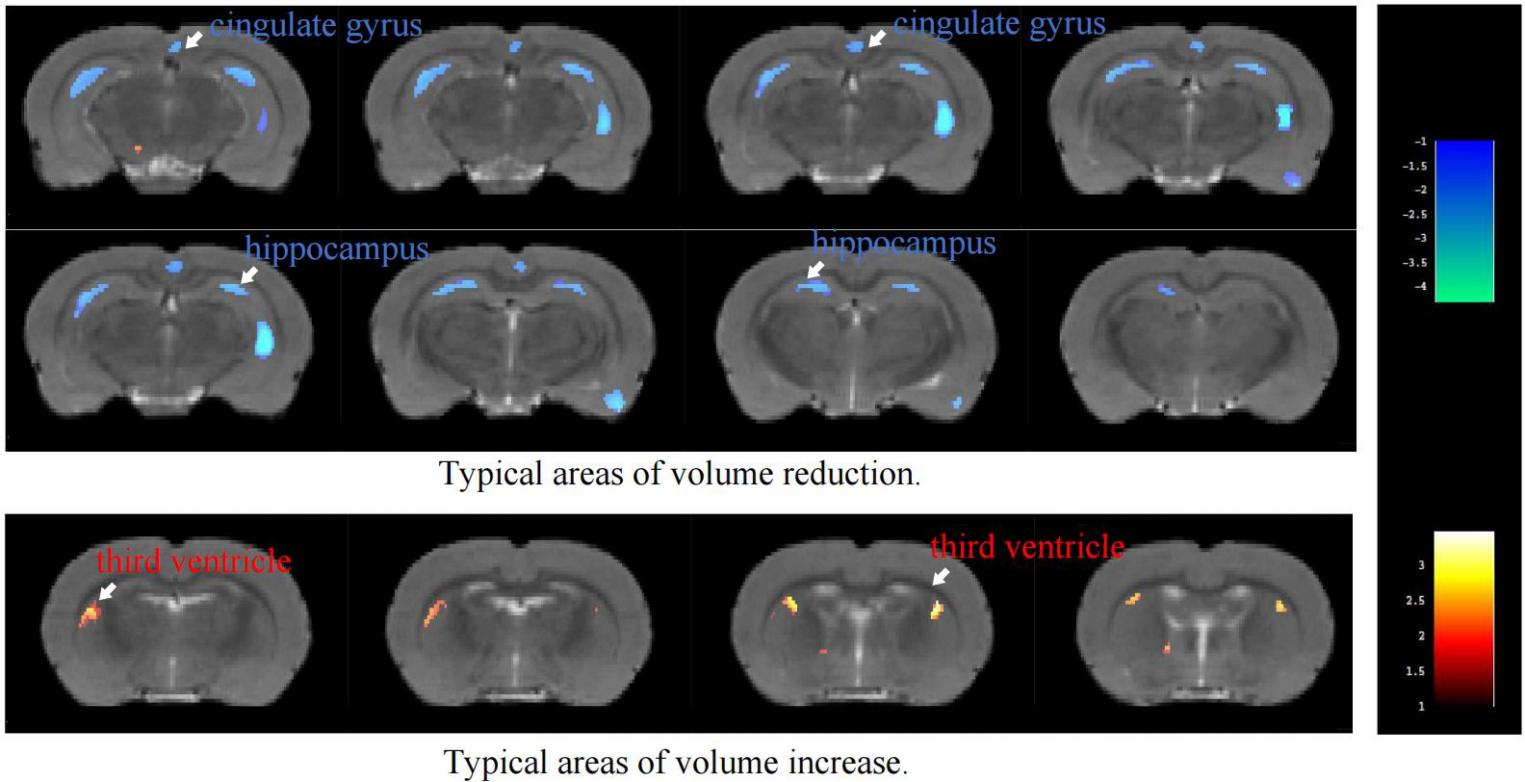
Color-coded VBM overlay images are displayed on the MRI reference map, with warm colors indicating increases and cool colors indicating decreases.
